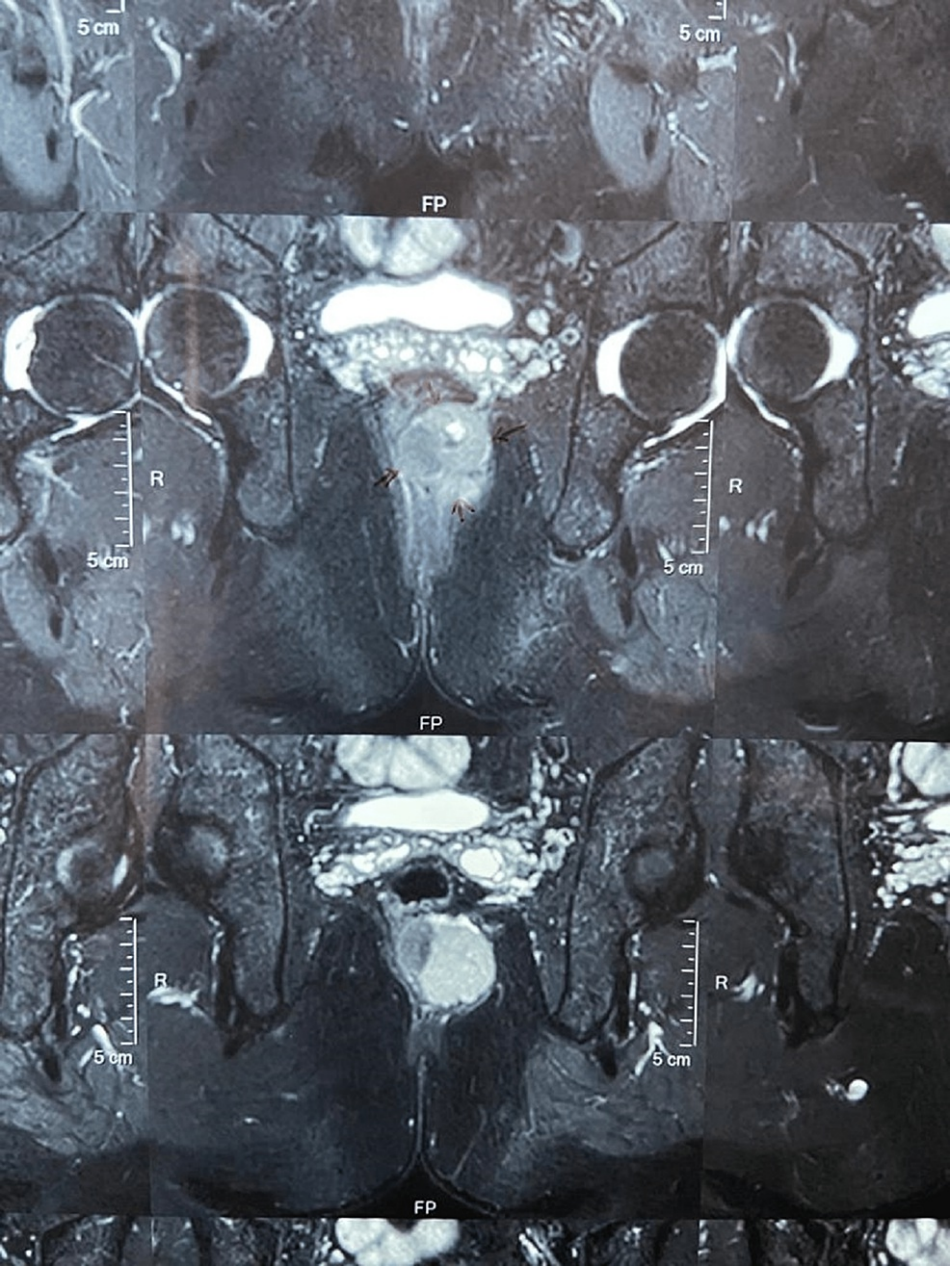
MRI indicating a well-circumscribed, solid, multilobulated lesion in the anal region on the left side with relation to the sphincter complex and projecting into the supralevator space.
